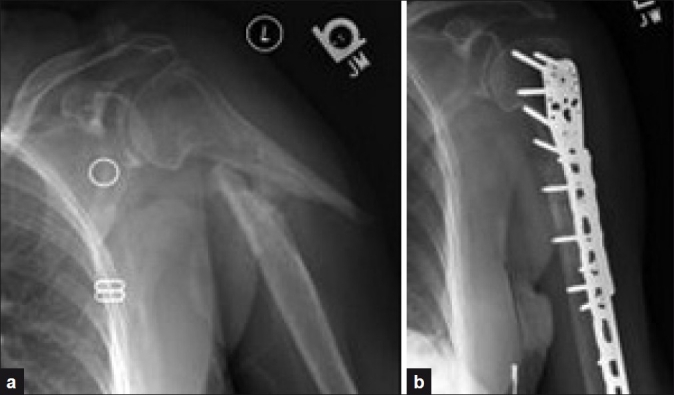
(a) Pre- and (b) post-operative radiographs of patient #1
